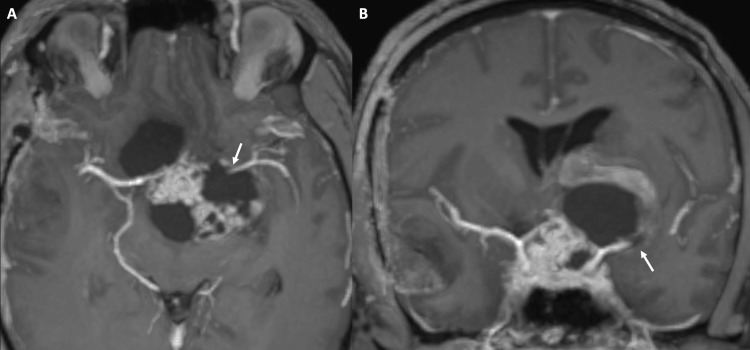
Axial and coronal sections of post-contrast T1-weighted maximum intensity projection images showing the lesion abutting the M1 segment of the left middle cerebral artery (arrows in A, B).
